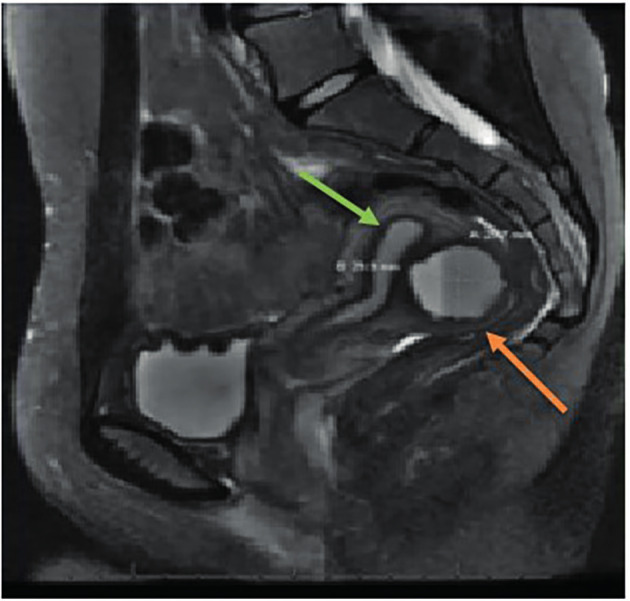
Magnetic resonance imaging picture of a juvenile cystic adenomyosis in the posterior wall of the uterus (orange arrow) of the same patient in Figure 1. The picture is showing the endometrial strip separate from the cyst (green arrow).
